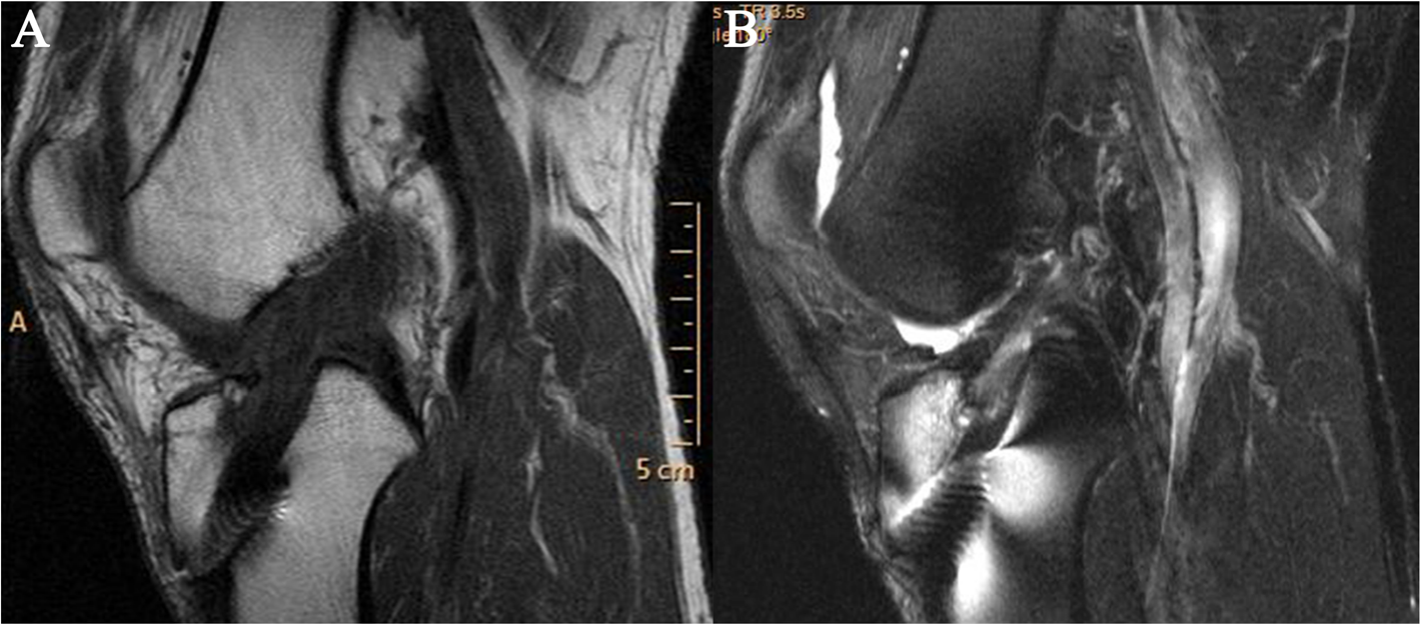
Preoperative MRI showed that the tibial tunnel was enlarged on the T1 sequence (a); on the T2-weighted images, there was a large effusion on both the femoral and tibial sides (b)
